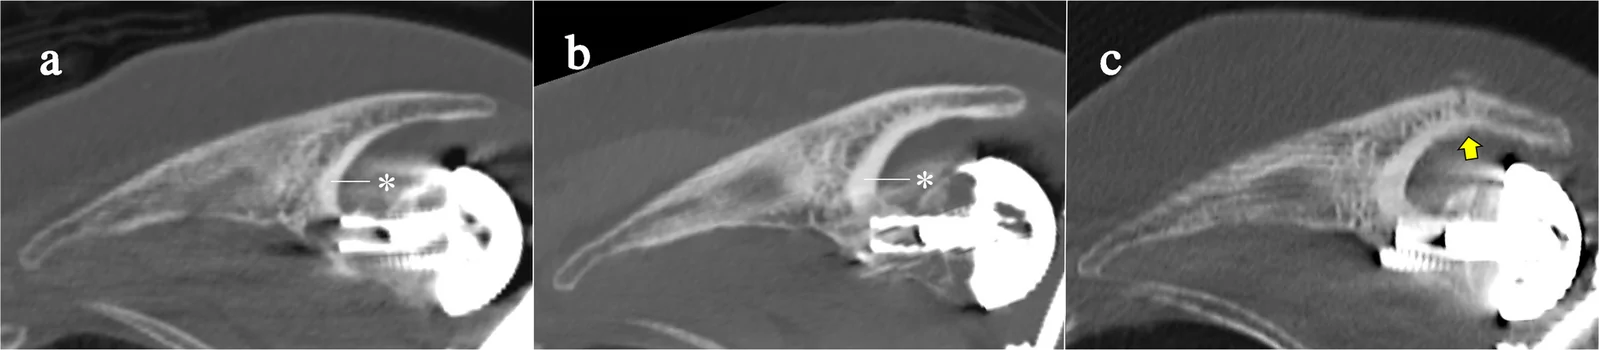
Representative case of delayed Zone II acromial fracture preceded by cortical thickening. CT-based acromial coronal views obtained immediately (a), 1 year (b), and 2 years post-operatively ^*^ (c) demonstrate progressive inferior cortical thickening in the corresponding zone before fracture detection (asterisks). Two years post-operatively, a fracture line was identified at the site of the preceding cortical thickening (yellow arrow). * The fracture-detection CT image was reconstructed with a 2-mm slice thickness and used for descriptive assessment only
